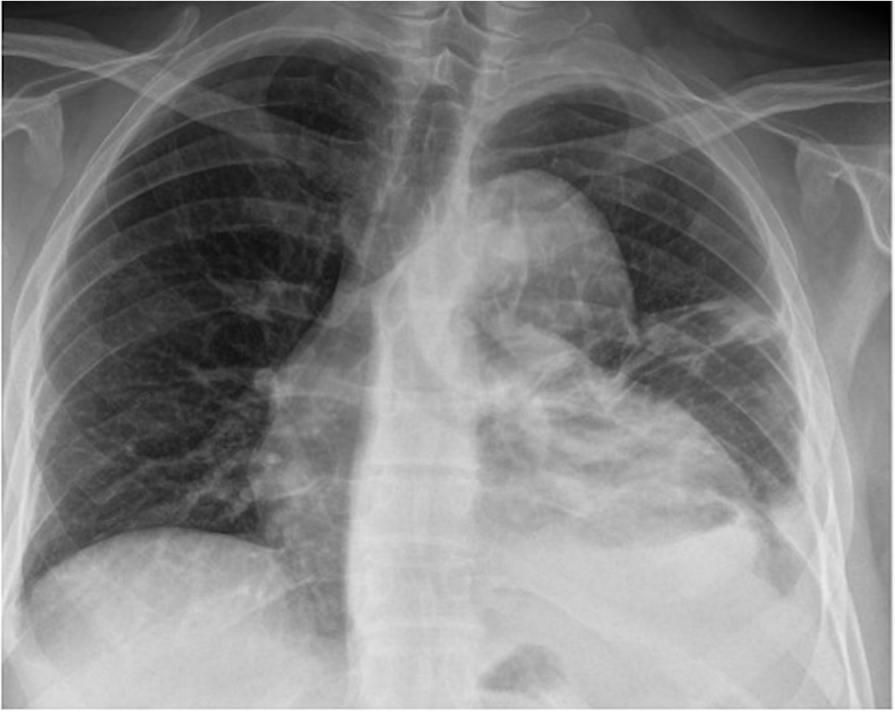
Chest radiograph demonstrating a left upper peri-mediastinal mass-like finding, with a left pleural effusion
